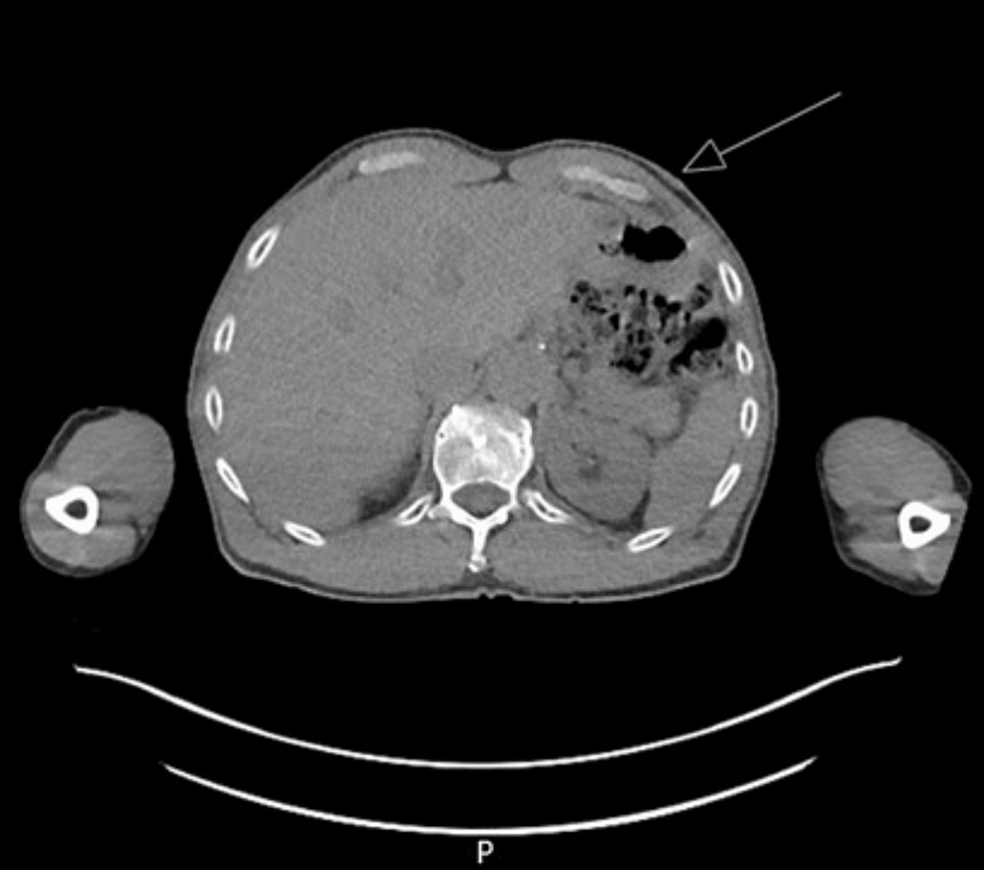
A CT PET from the skull base to mid-thigh, May 2021 Moderate hypermetabolic activity is associated with the high left axillary biopsy-proven malignancy. A second, more medially located hypermetabolic lymph node in the left upper chest, subpectoral region, is suspicious for metastasis as well. There is no definite linear extension along the neurovascular bundle.
